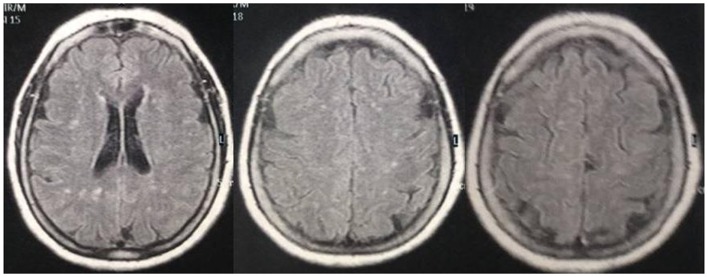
MRI of a 69-year-old woman with a long history of migraine attacks. Note the white matter abnormalities, frequently encountered in migraineurs.
